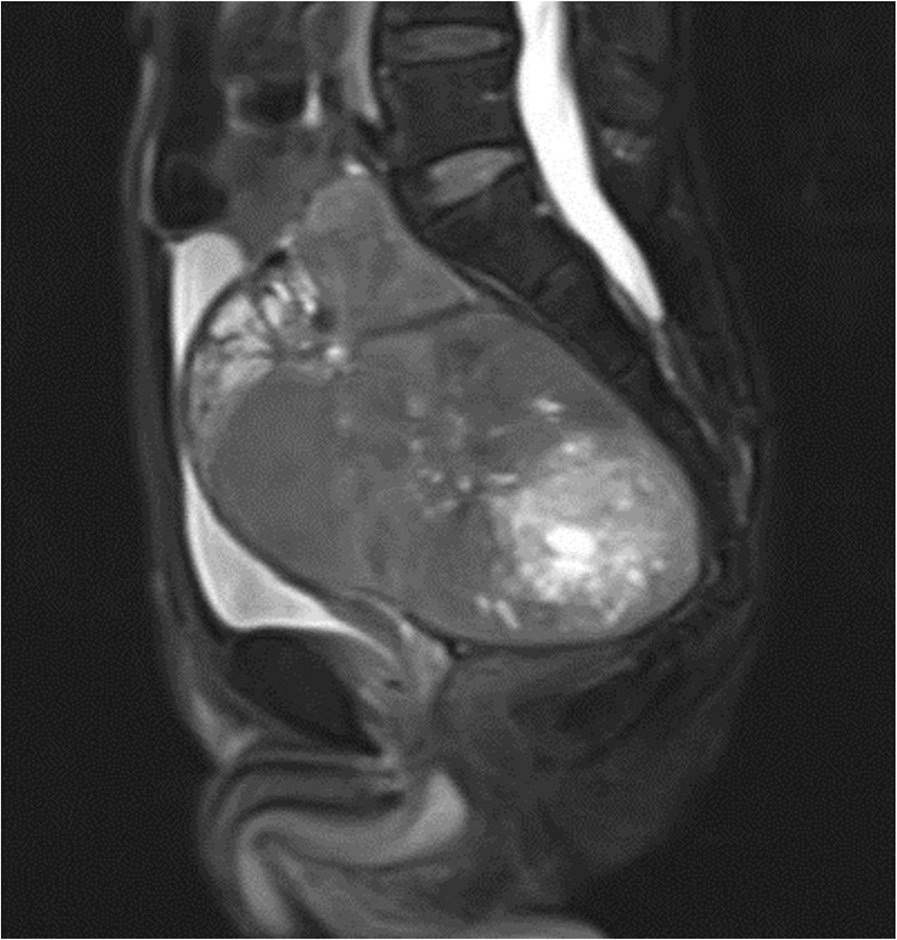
T2-weighted pelvic MRI image. No clear invasion to any adjacent organs is identified
